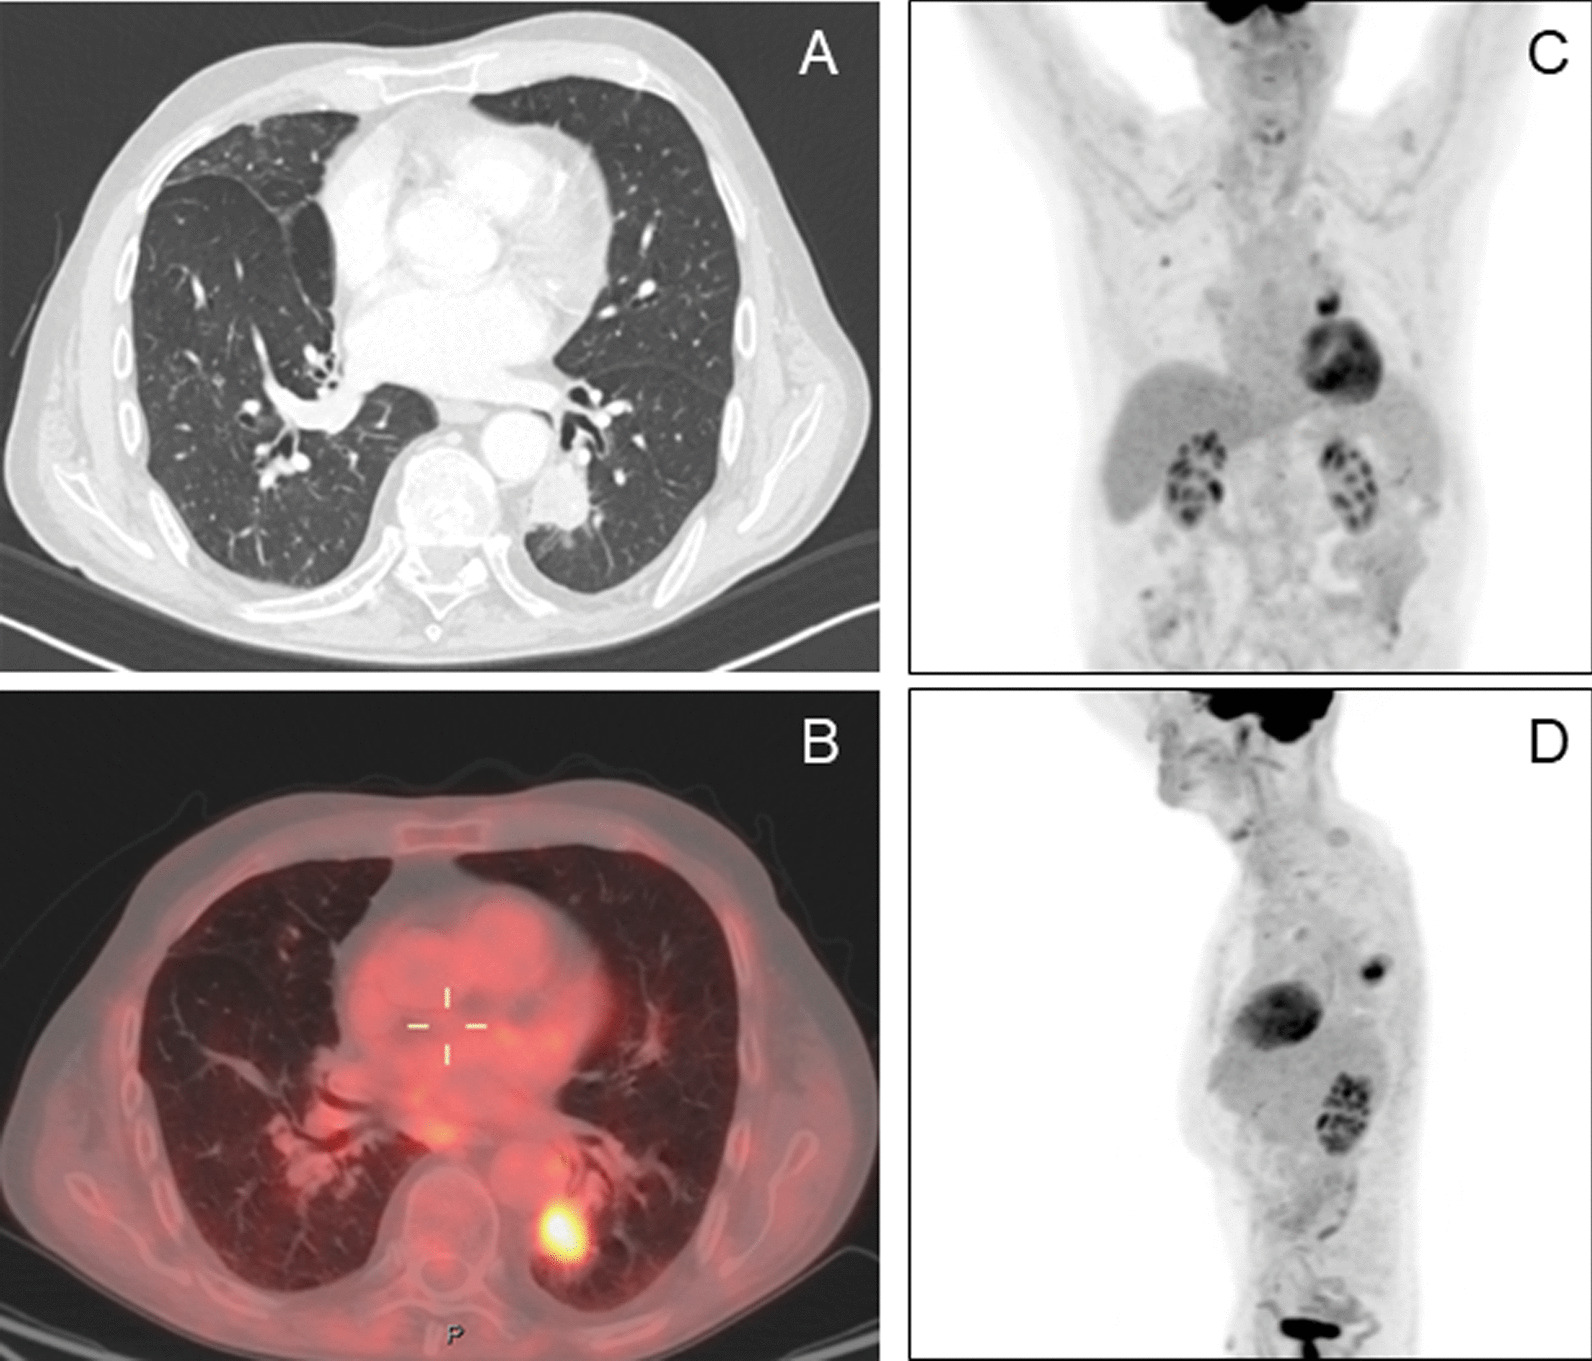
Chest CT scan and FDG PET-CT. A Chest CT scan in axial plane. B FDG PET-CT in coronal plane. C FDG PET-CT in sagittal plane. D Chest CT scan and FDG PET-CT in axial plane
